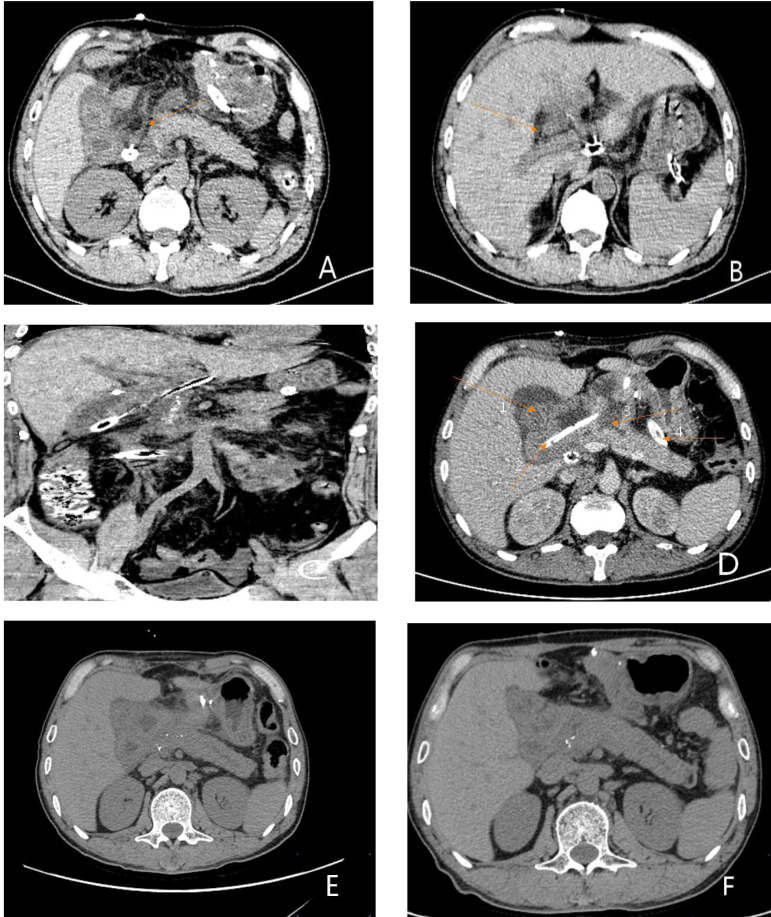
Postoperative CT results. (A) Plain CT scan of the whole abdomen on the 3rd postoperative day. The arrow shows the pancreaticojejunostomy. (B) Full abdominal CT reexamination on day 3 postoperatively. The arrow showed the pancreaticojejunostomy. (C) Full abdominal CT reexamination on day postoperatively showing the entire pancreaticoduodenectomy area. (D) Plain CT scan of the whole abdomen on day 11 postoperatively. Arrow 1 shows the biliary and intestinal anastomosis, arrow 2 shows the indwelling open-field decompression tube intraoperatively, arrow 3 shows the pancreaticojejunostomy, and arrow 4 shows the indwelling three-cavity irrigation and drainage tube intraoperatively. (E) Plain CT scan of the whole abdomen 1 month after surgery showing no obvious abnormalities. (F) Plain CT scan of the whole abdomen 3 months after surgery indicating good recovery.
